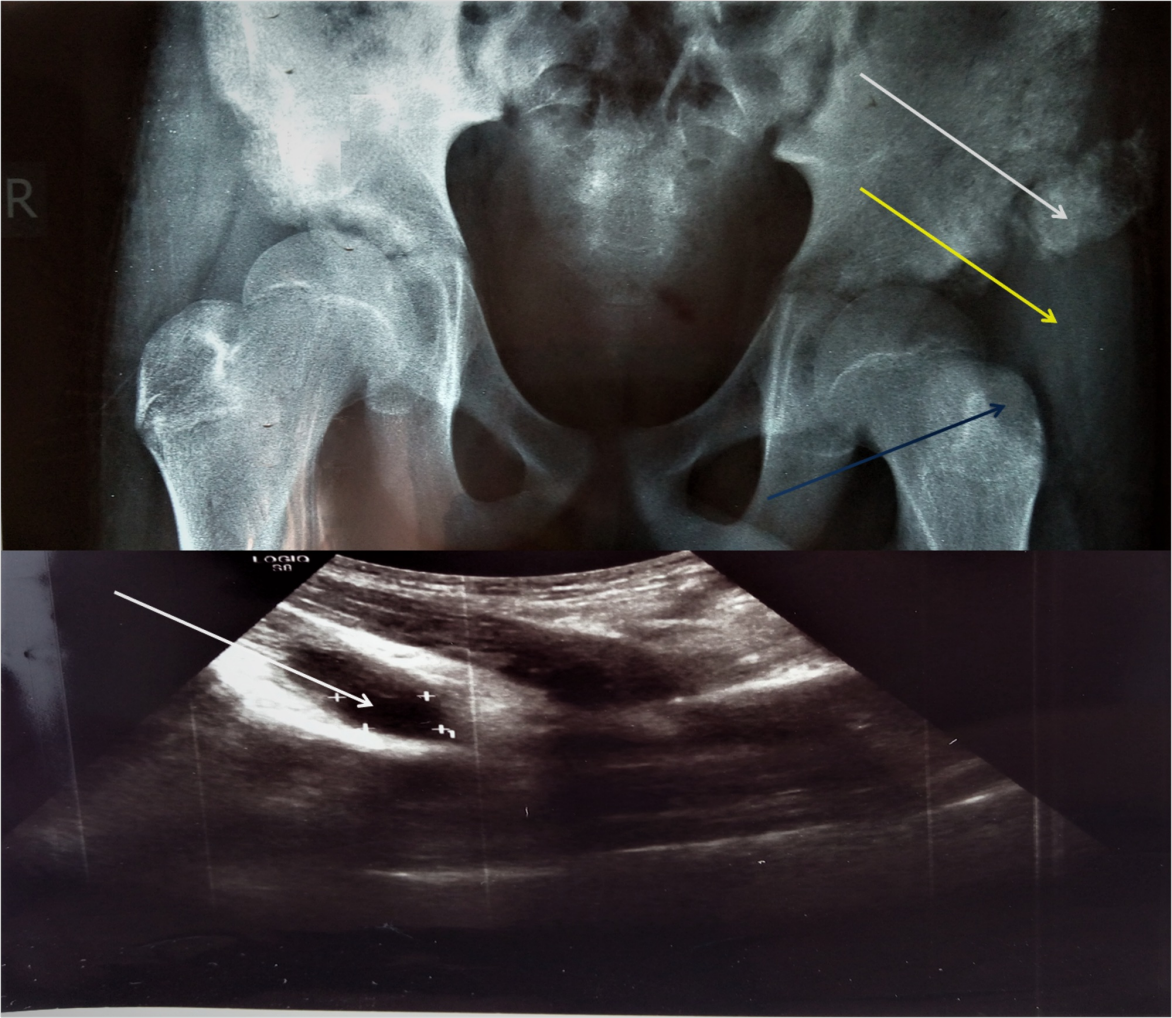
Radiograph and ultrasonography (USG) of patient No. 2. Calcification of a cystic formation (abscess) next to the left iliac wing (white arrows). USG report mentions a cystic formation (abscess) inside the left psoas muscle with dimensions of 3.94 cm x 2.01 cm. Swelling of soft tissue over the left trochanteric area (yellow arrow). Lytic lesion on the left trochanter (blue arrow).
